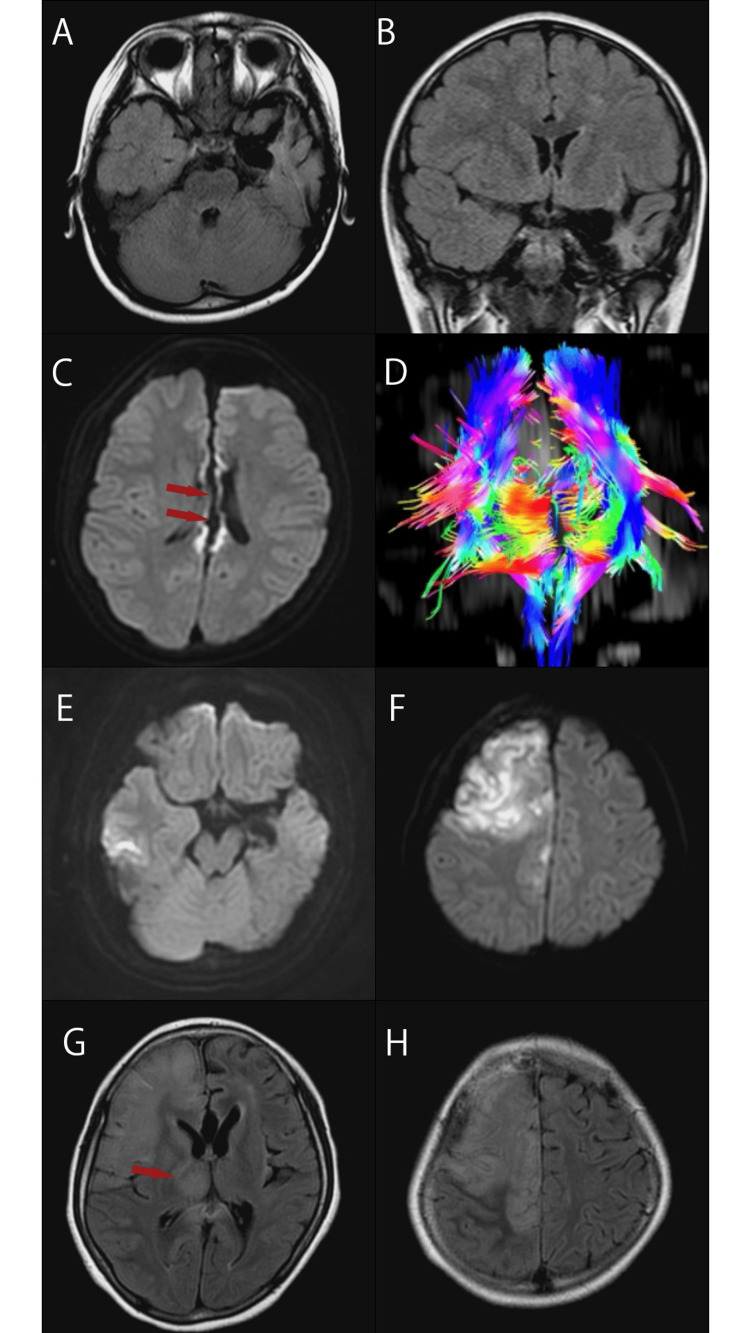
MR images are shown in chronological order MR: magnetic resonance; POD: postoperative day; FLAIR: fluid-attenuated inversion recovery After the initial HSE, lesions remained from the lateral left temporal lobe to the insular cortex (A: axial FLAIR image, B: coronal FLAIR image). Post-corpus callosotomy MR images show minimal impact on surrounding areas (C: axial diffusion image, arrowhead; D: tractography by diffusion tensor imaging). The MR image on POD 10 shows no abnormalities in the initial lesion site of the left temporal lobe (E: axial diffusion image), but a lesion is observed as a hyperintense area in the right frontal lobe (F: axial diffusion image). On the MR image of POD 23, the lesion has expanded to the entire frontal lobe (G: axial FLAIR image, H: axial FLAIR image), and it is evident that the lesion extends to the thalamus (arrowhead)
